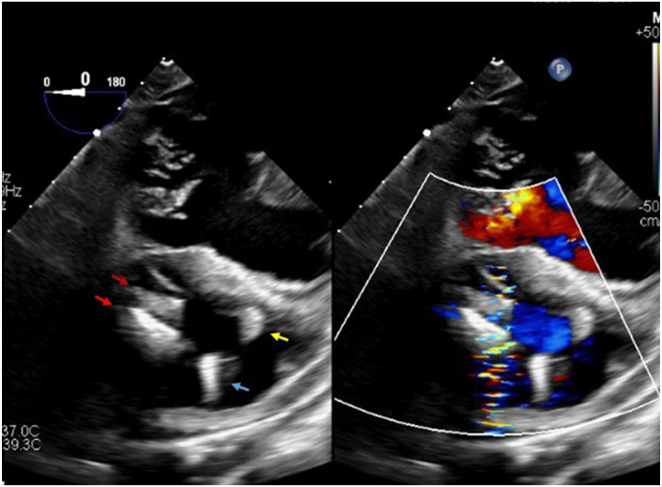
TEE mid-esophageal view at 0 degree. *Red arrows* showed TV clips, *blue arrow* showed part of depleted Micra VR, and *yellow arrow* showed part of AVEIR VR after deployment. TEE = transesophageal echocardiography; TV = tricuspid valve.
